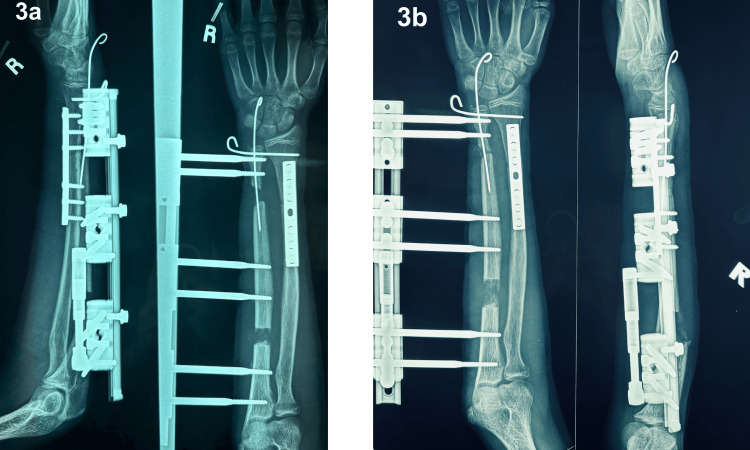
Radiographs of the right radius and ulna during the distraction phase, on day 10 (3a) and day 20 (3b), showing gradual bone transport and healing progress.
